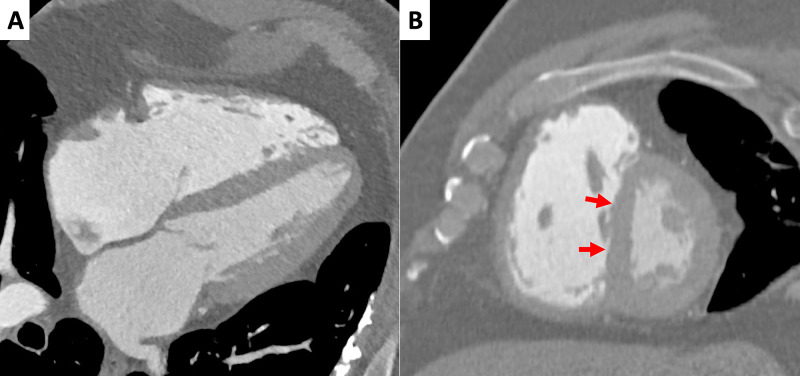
Cardiac computed tomography: functional and morphological evaluation (A,B) Horizontal long axis and short axis plane, respectively; 80% R-R interval. The images confirm right atrium and ventricle dilation, with hypertrophic appearance of the latter, as seen before in cardiac magnetic resonance scans. Furthermore, the left ventricle appears «D-shaped» due to interventricular septum flattening (arrows).
